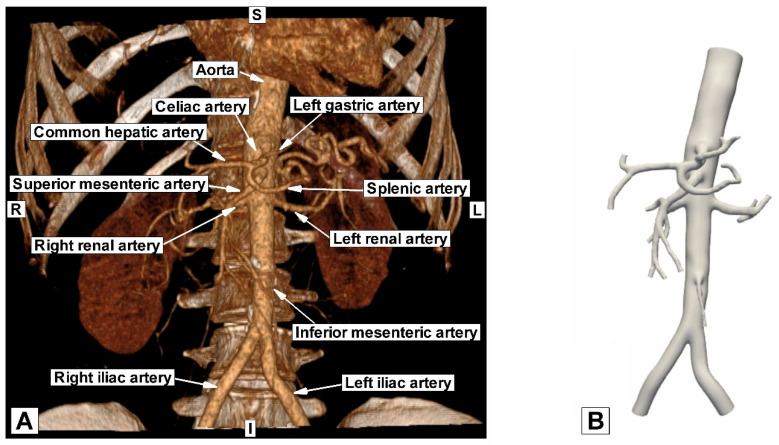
Comparison between geometry reconstruction using (A) 3D VRT (volume rendered technique) based on CTA images; and (B) advanced numerical techniques.
